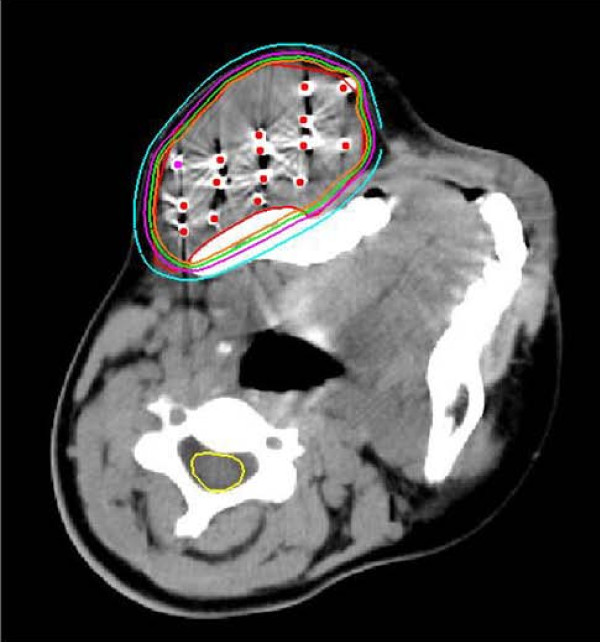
The isodose curve after seed implantation from CT scan. The inner red cure represents GTV. The ellipses are iso-dose lines of 160, 140, 120, 90 Gy from inside, respectively.
